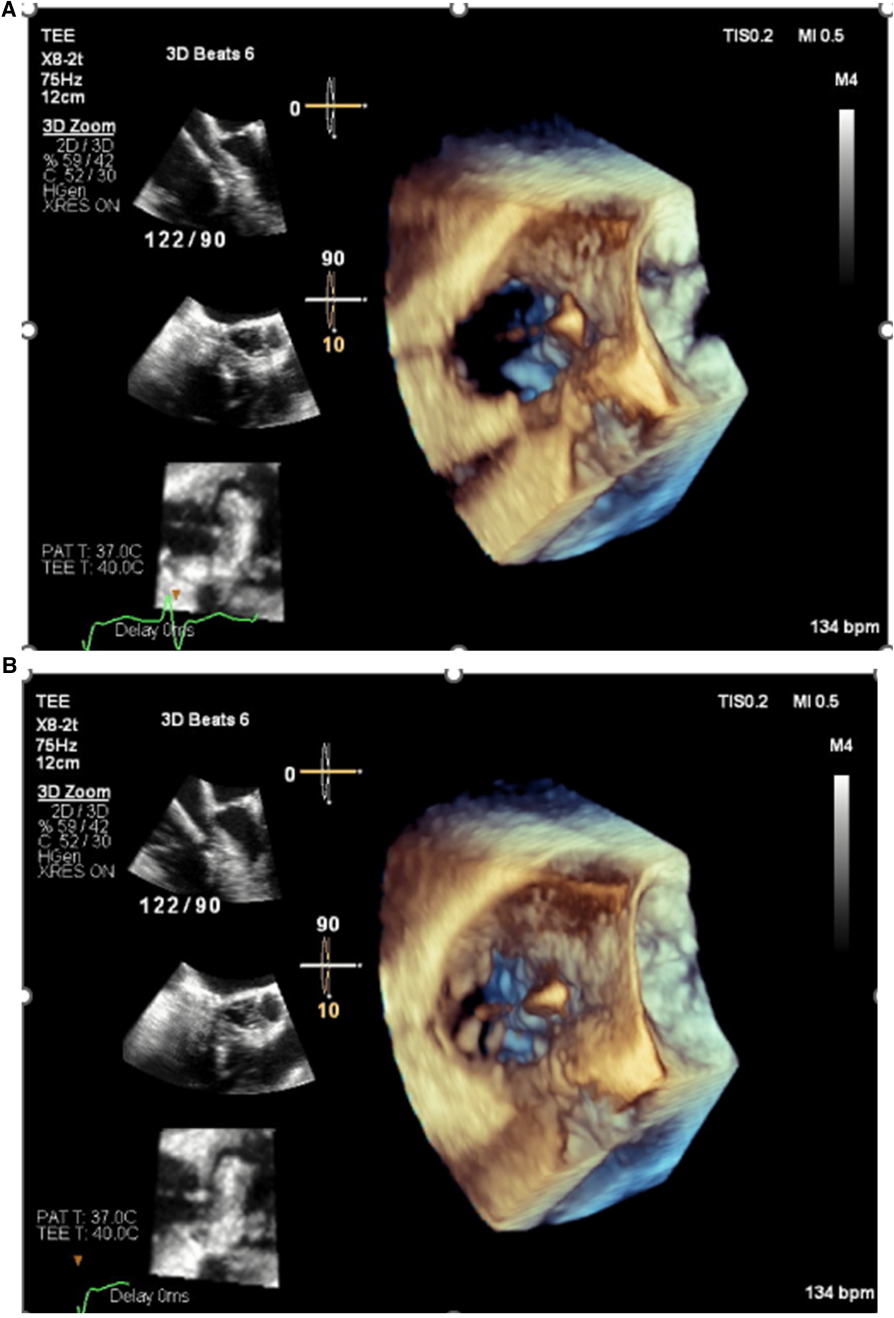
Echocardiogram images demonstrating pinning of the septal leaflet of the tricuspid valve to the septum by a pacemaker lead resulting in severe tricuspid regurgitation. (A) Valve open in diastole. (B) Valve closed in systole.
